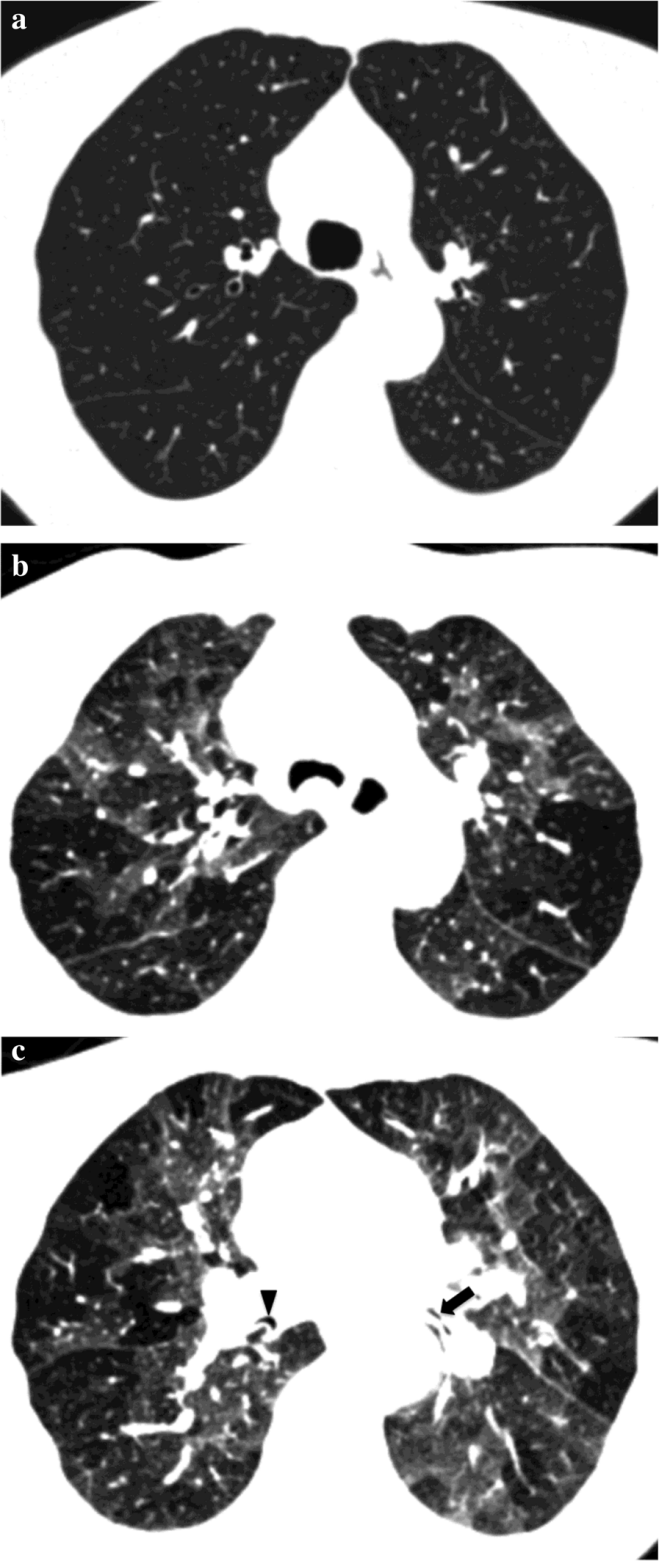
64-year-old man with tracheobronchomalacia complaining of chronic cough, exertional dyspnea and wheezing. Inspiratory axial CT scan through upper lobes shows normal trachea and lung (a). Expiratory axial CT scan demonstrates collapse of trachea due to tracheobronchomalacia as well as extensive air-trapping (b). On axial expiratory CT scan at subcarinal level collapse of the intermediate (arrowhead) and lower lobar (arrow) bronchi due to bronchomalacia can be seen (c). Note extensive air-trapping of the lower lobes.
